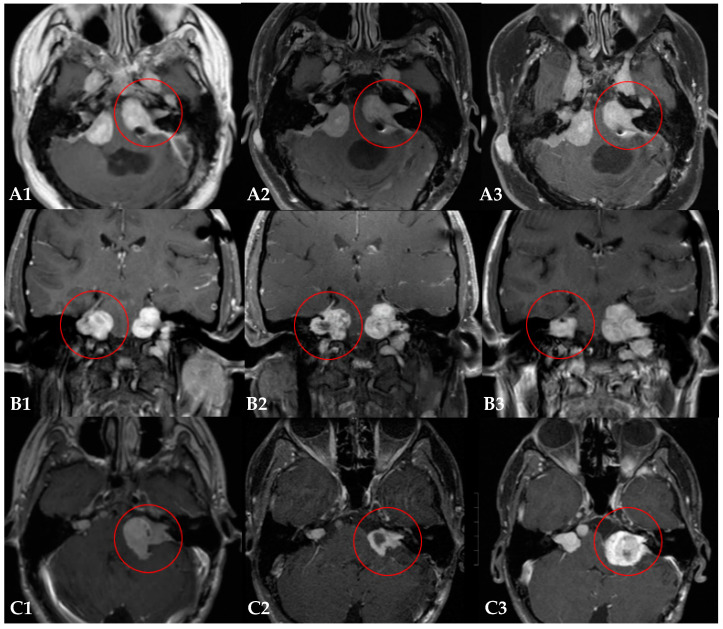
Three examples of the radiological follow-up of Neurofibromatosis Type 2-related vestibular schwannomas treated with proton radiotherapy (PRT). Each row (A–C) represents MR imaging of one patient over time: the baseline MR image (1), the MR image one year after PRT (2), and the most recent MR image (3). Patient (A): the tumor of interest remained stable during the follow-up of 60 months (A1–A3). Patient (B): the initial tumor shrinkage achieved with PRT and adjuvant bevacizumab therapy (B1), was followed by tumor progression (B2) that required additional treatments with bevacizumab (B3). Patient (C): the initial tumor shrinkage (C1,C2) was followed by significant tumor progression (C3), requiring salvage surgery at 87 months after PRT.
